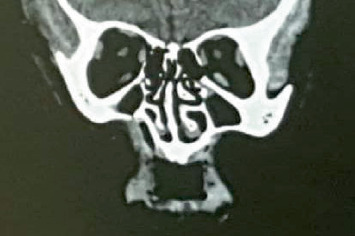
CT scan for the head shows the small hole in the floor of the maxillary sinus in the right side.
